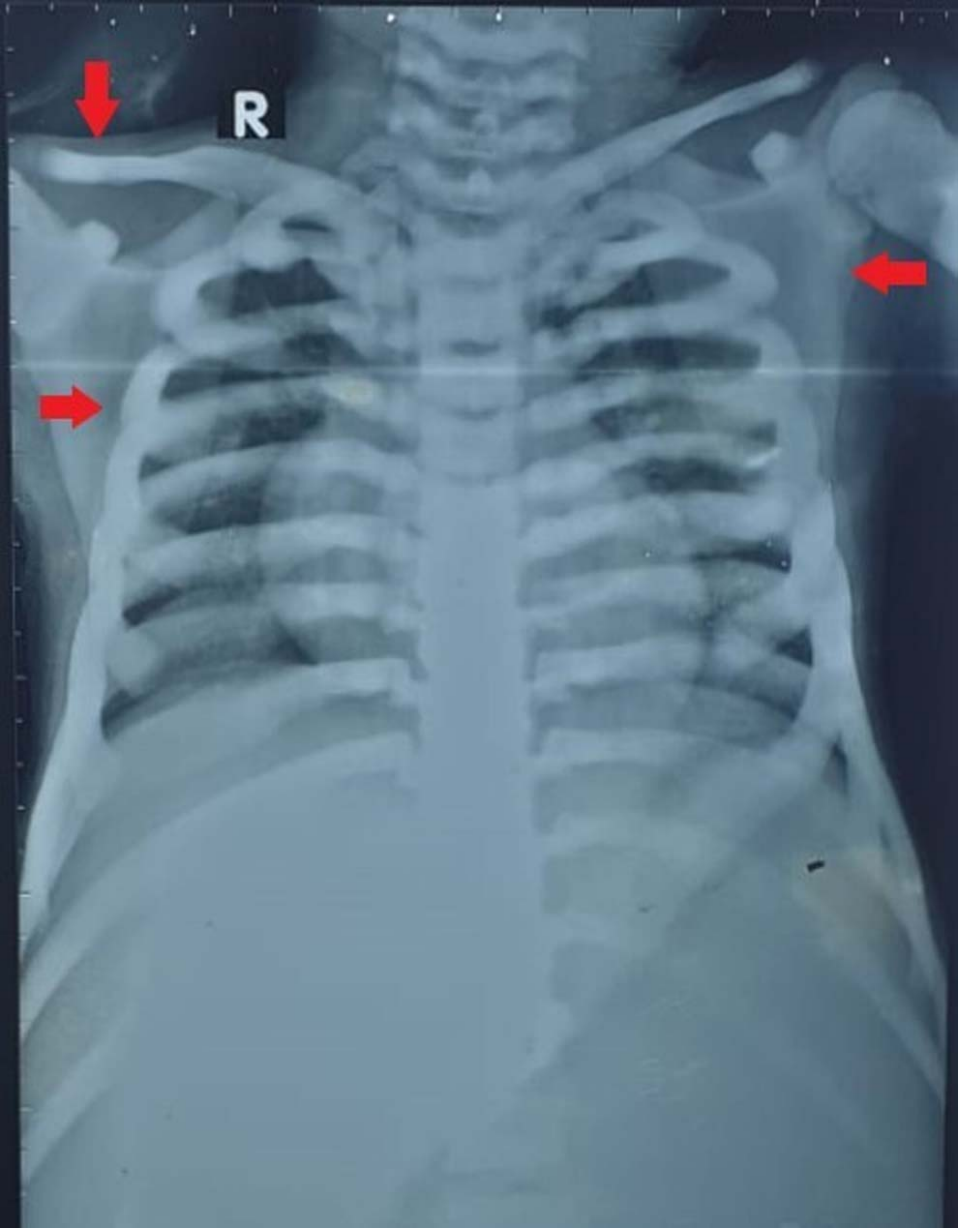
Chest x-ray shows a generalized increase in bone density in the clavicles, scapulae, and ribs (arrows).
